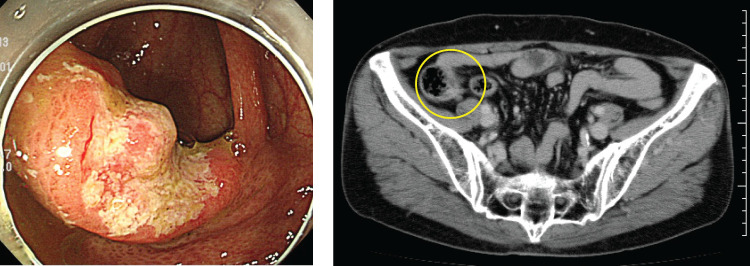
Left panel: Colonoscopy images are shown. Right panel: One slice of contrast CT images is shown. The yellow circle indicates the lesion.
